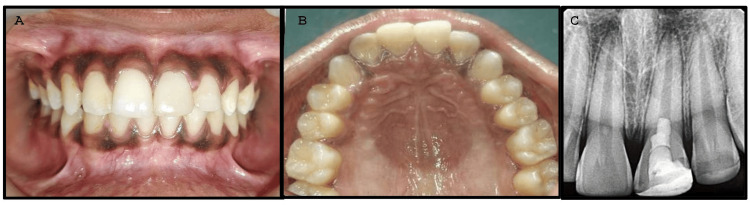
Clinical and radiographic pictures after one year of follow-up
